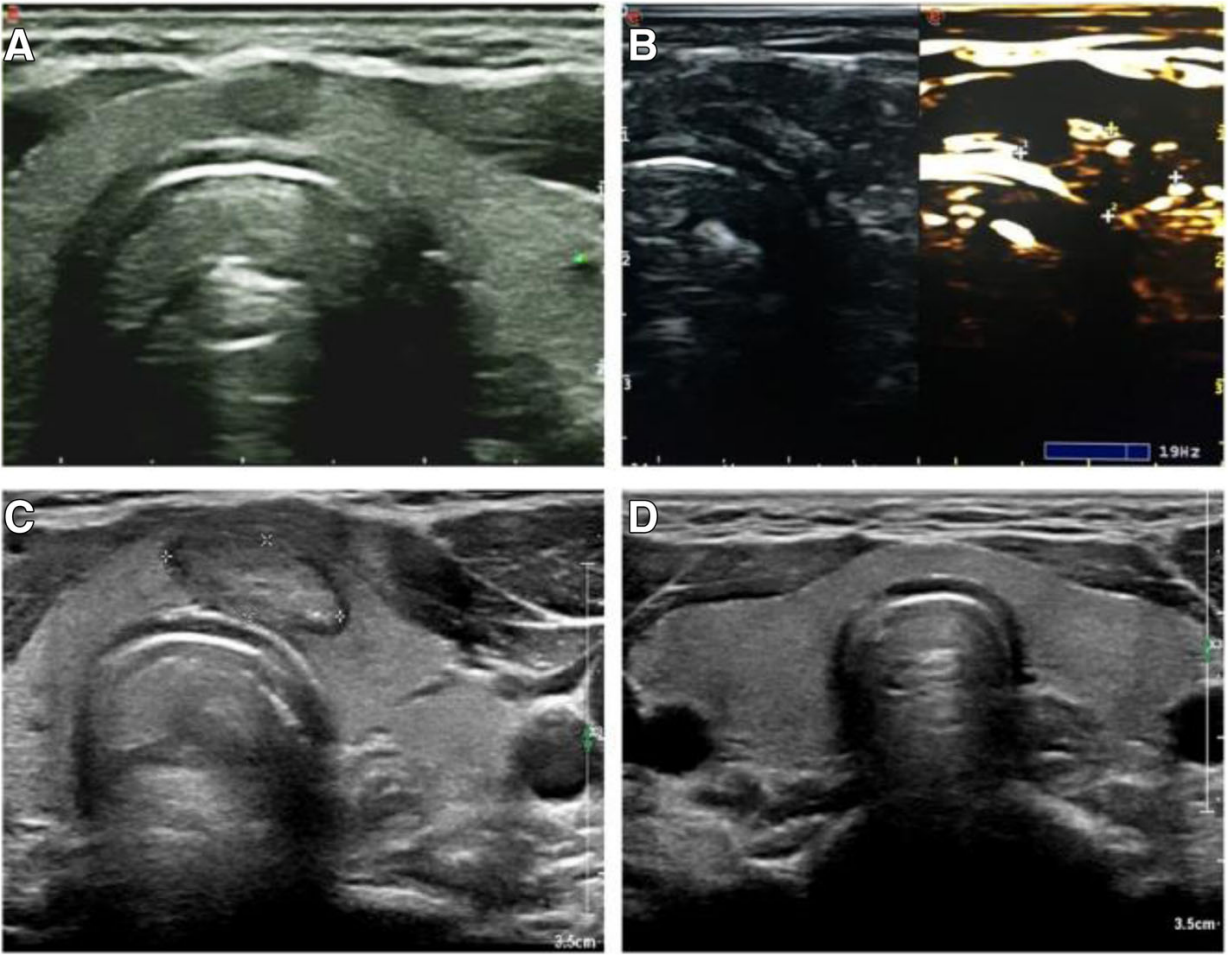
a 66-year-old male with a PTMC lesion near the trachea in whom conventional ultrasound examination showed a 9.7 mm × 8.4 mm × 5.5 mm hypoechoic nodule. b After PLA, CEUS revealed a hypoechoic area in the original area, and some debris in the centre presented as strong echoes, which demonstrated that the lesion had been completely ablated. c One month after PLA treatment, conventional ultrasound examination showed a 15.1 mm × 6.2 mm × 3.3 mm hyperechoic nodule. d Eighteen months after PLA treatment, conventional ultrasound examination showed that the lesion had completely disappeared
